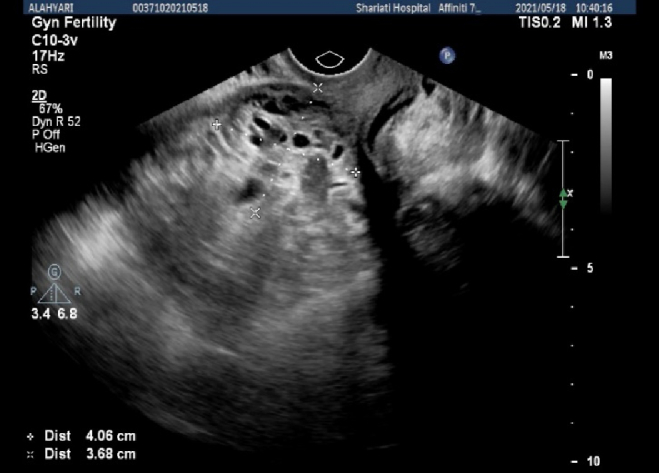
Transvaginal ultrasonography (a heterogeneous thick area containing cystic changes with a size of 59
